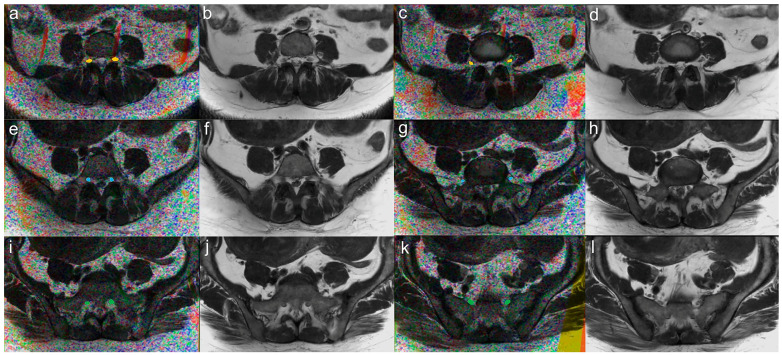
Axial view of fusion (a,c,e,g,i,k) and T2-weighted (b,d,f,h,j,l) images from a DPN patient (54-year-old female). ROI placement for measurement of ADC and FA. The ROIs of seeding points were placed at two levels of one nerve root in the fusion images: one was at the level of middle spinal body (a,e,i) and another was at the level of inferior spinal disc (c,g,k). Yellow ROIs represent the L4 nerve roots; blue ROIs represent the L5 nerve roots; and green ROIs represent the S1 nerve roots.
